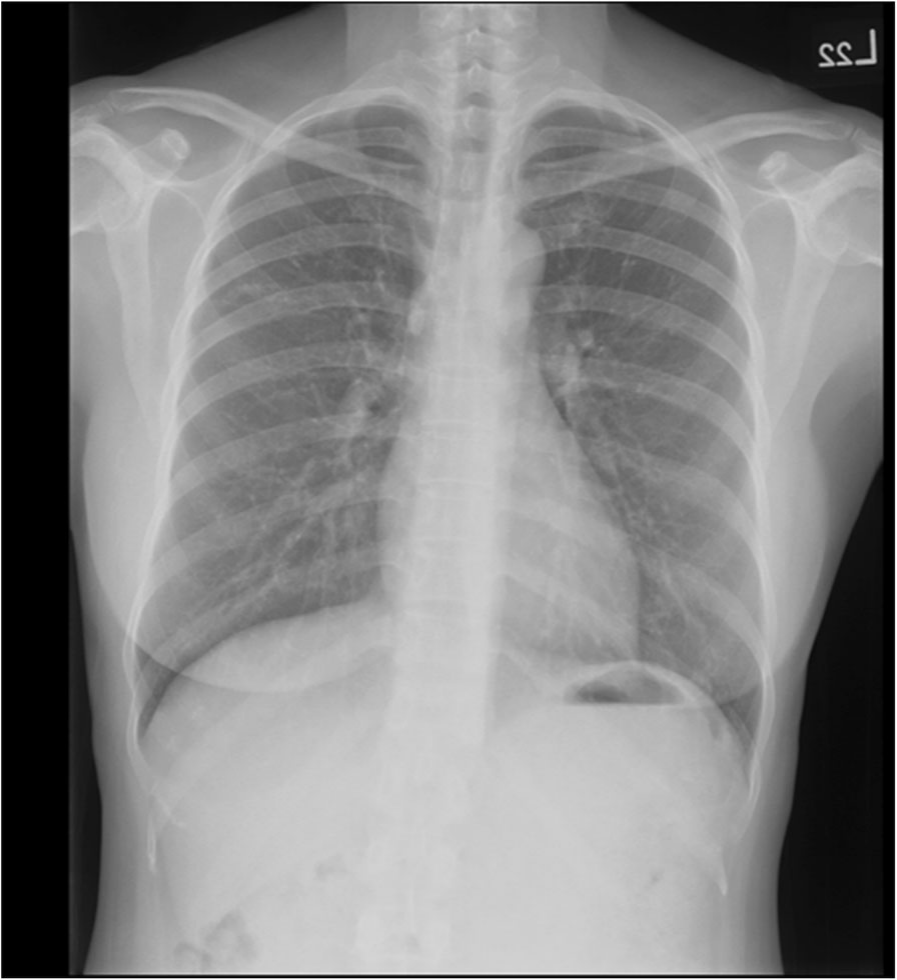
Follow-up chest radiograph obtained 2 years later. Two years later, a chest radiograph showed a change in the shape and decrease in the size of the calcified nodule in the upper right lobe (yellow arrowhead)
